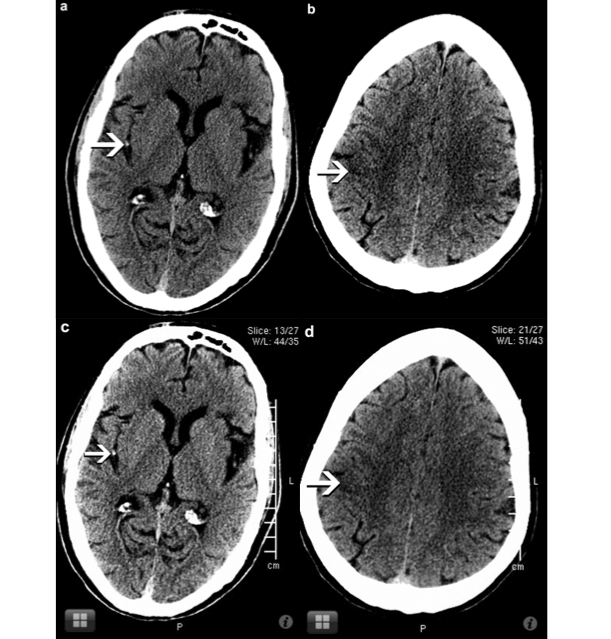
In image *a*, the workstation monitor images show a dense MCA sign (arrow) and in image *b*, acute ischemic change in the ASPECTS M6 region (arrow). Corresponding iOS device images show a dense MCA sign (arrow) in image *c* and acute ischemic change in ASPECTS M6 region (arrow) in image *d*.
